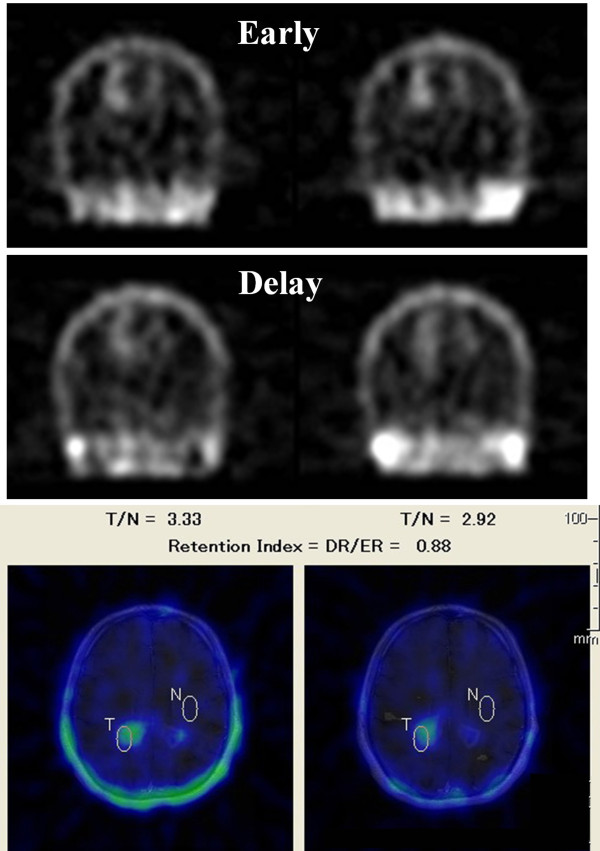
Uptake of thallium in the lesions on ^201^Tl scintigraphy. Definite uptake of thallium is identified in the lesions. However, the retention index (ratio of thallium uptake between late and early phases) is 0.88, suggesting a low possibility of malignancy.
